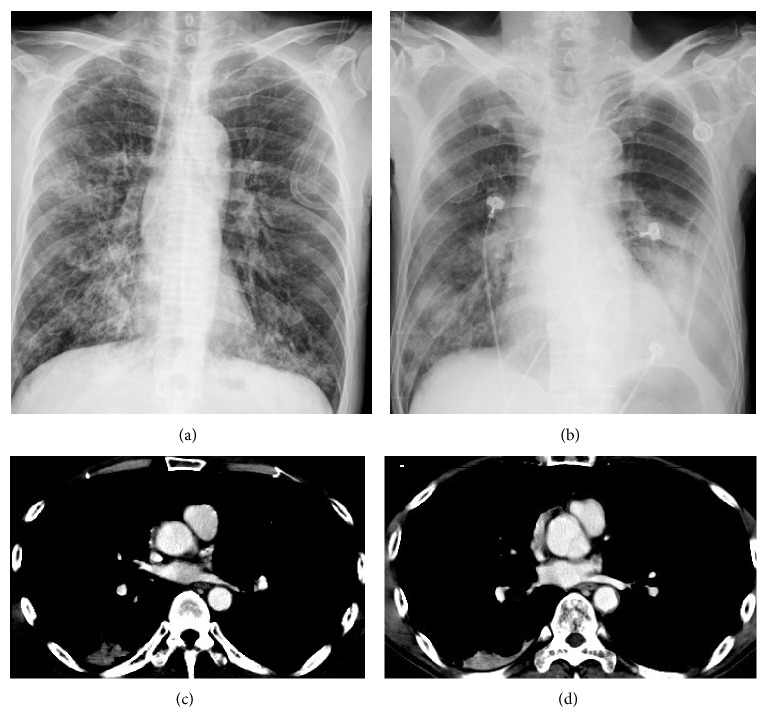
Pulmonary vein obstructive syndrome (PVOS). A 51-year-old man with oral cancer. Chest X-ray (a) before and (b) at the onset of PVOS showed a left low lung and hilum increase haziness. CT scan ((c) and (d)) revealed tumor/thrombosis located in superior and inferior pulmonary veins with left atrium extension.
